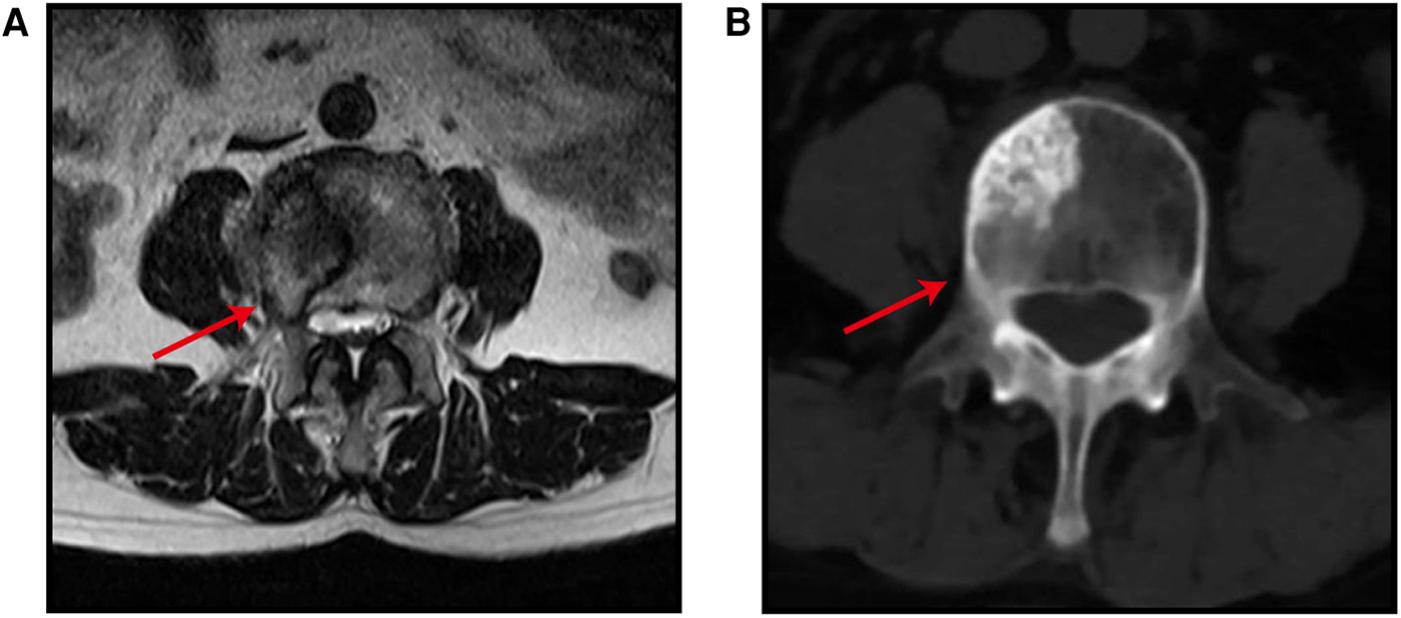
MRI image (A) and CT image (B) show metastatic tumor involve unilateral articular joints and cause nerve root involvement and mild epidural compression. The red arrow points to the location of the lesion.
